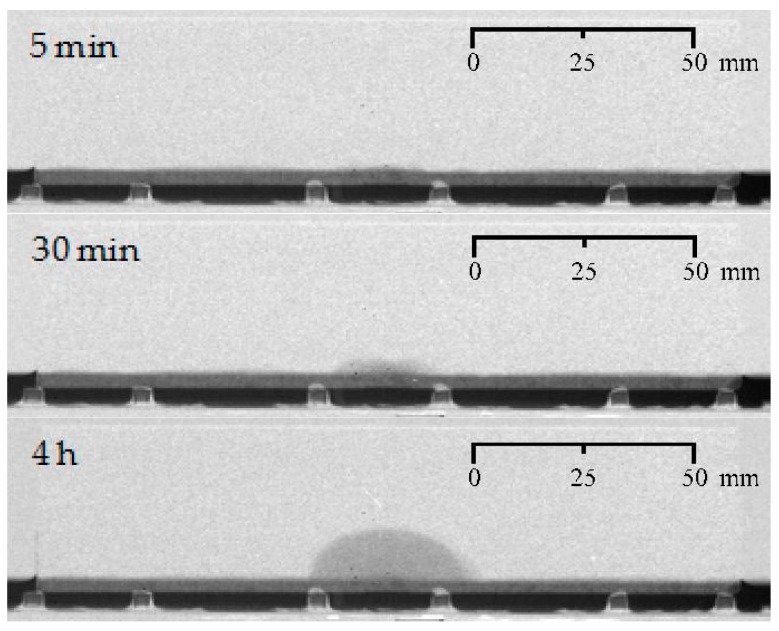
Visualization of the water uptake as a function of exposure time using neutron radiography for one uncracked mortar prism (representative sample: UN_A).
